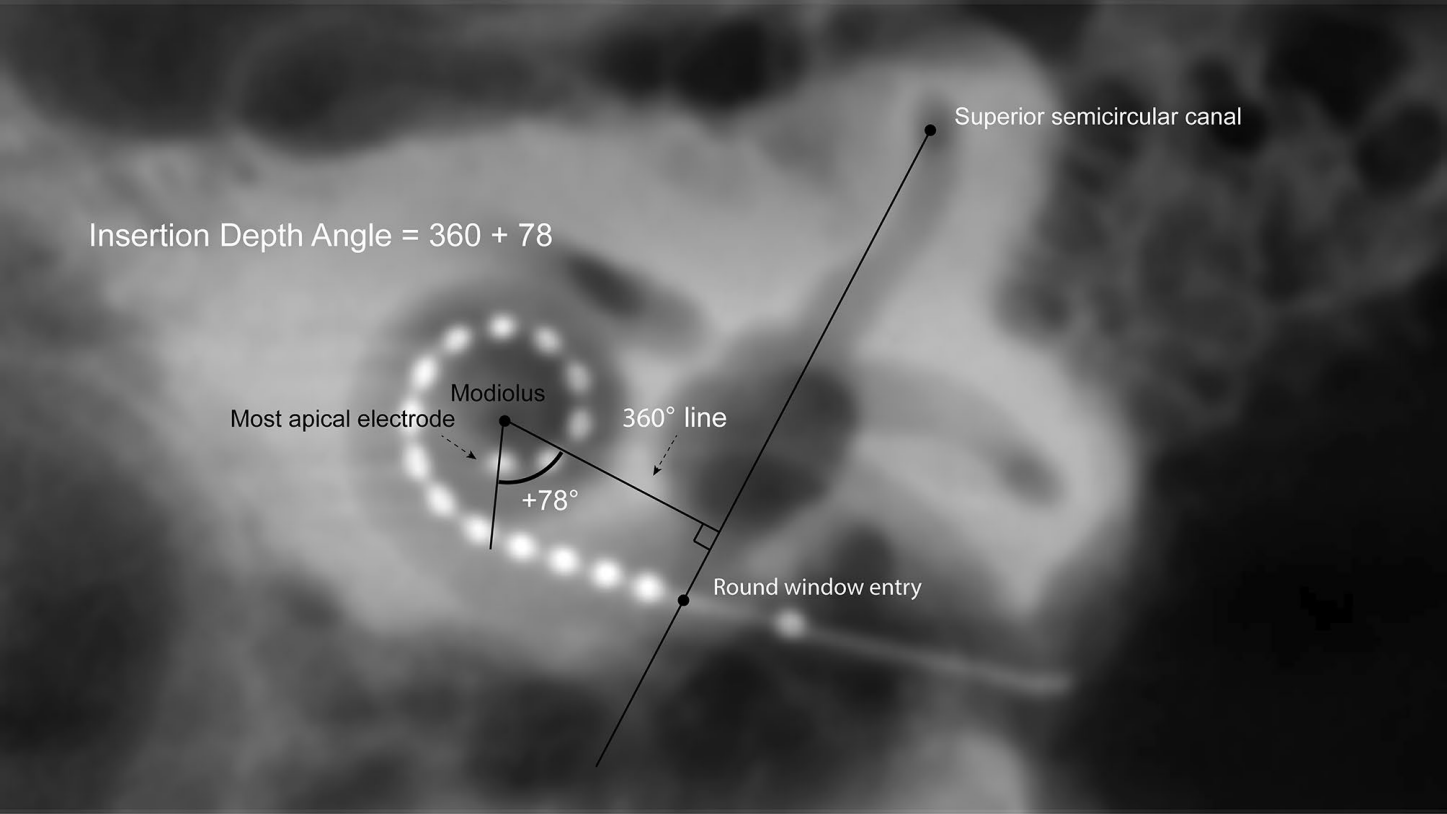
In the cochlear view reconstruction of CB-CT scan both insertion depth angle and distance A can be measured. The 360° line is drawn perpendicular to a line between round window entry and middle of upper part of the posterior semicircular canal. The insertion depth angle is measured by adding 360° to the angle between the apical electrode and the 360° line. Distance A (dashed line), an indirect measure proportional to cochlear duct length, is measured as the length of the line from the point of the array entering the RW or CO, through the modiolus to the contralateral cochlear wall.
